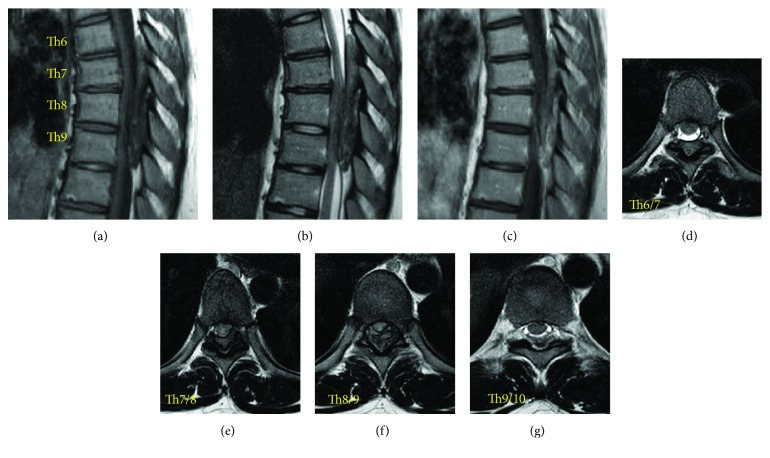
Preoperative thoracic MRI. Heterogeneous epidural posterior mass compressing the thoracic cord between Th6 and Th9 was revealed at the admission. Sagittal view of heterogeneous isointense mass of T1-weighted image (a), heterogeneous isointense mass of T2-weighted image (b), and nonenhancing mass of Gd-enhanced T1-weighted image (c). Axial view of epidural mass of T2-weighted image in Th6/7 (d), Th7/8 (e), Th8/9 (f), and Th9/10 (g) intervertebral levels. This epidural mass was considered as an old epidural hematoma initially.
